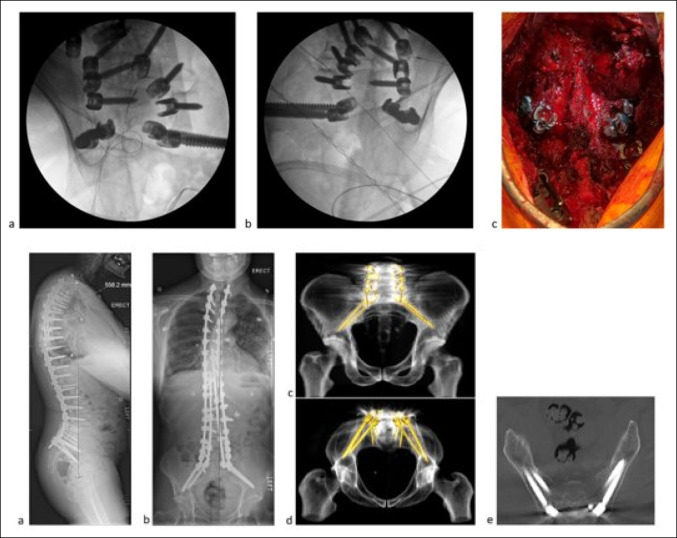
Top: Images showing intraoperative fluoroscopy views of the anatomic iliac screws and S2 pelvic screws, (A) left view, (B) right view, and (C) intraoperative photograph. Bottom: (A and B) Postoperative standing radiographs and CT scans showing quad-rod technique for sagittal and coronal correction. C and D, 3D reconstruction from CT scans demonstrating the placement of pelvic instrumentations. E, Axial CT scan demonstrating the trajectory of the iliac screws within the pelvic wings.
